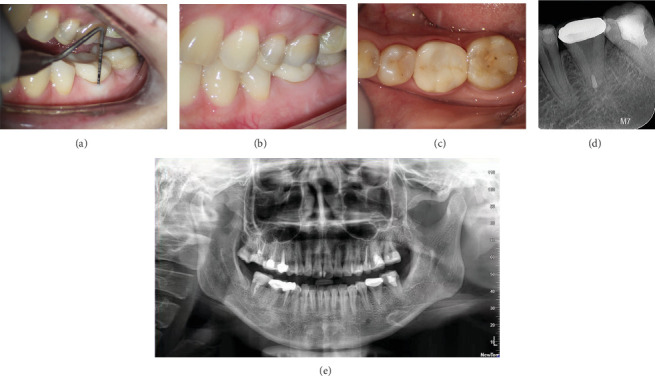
Clinical follow-up at 2 years. (a) The periodontal probing depth of the transplanted tooth was normal, which meant that tooth autotransplantation obtained excellent epithelial attachment. (b) The buccal view of the transplanted tooth. (c) The occlusal view of the transplanted tooth. (d) Periapical radiograph 2 years after transplantation. (e) Panoramic radiograph 2 years after transplantation.
